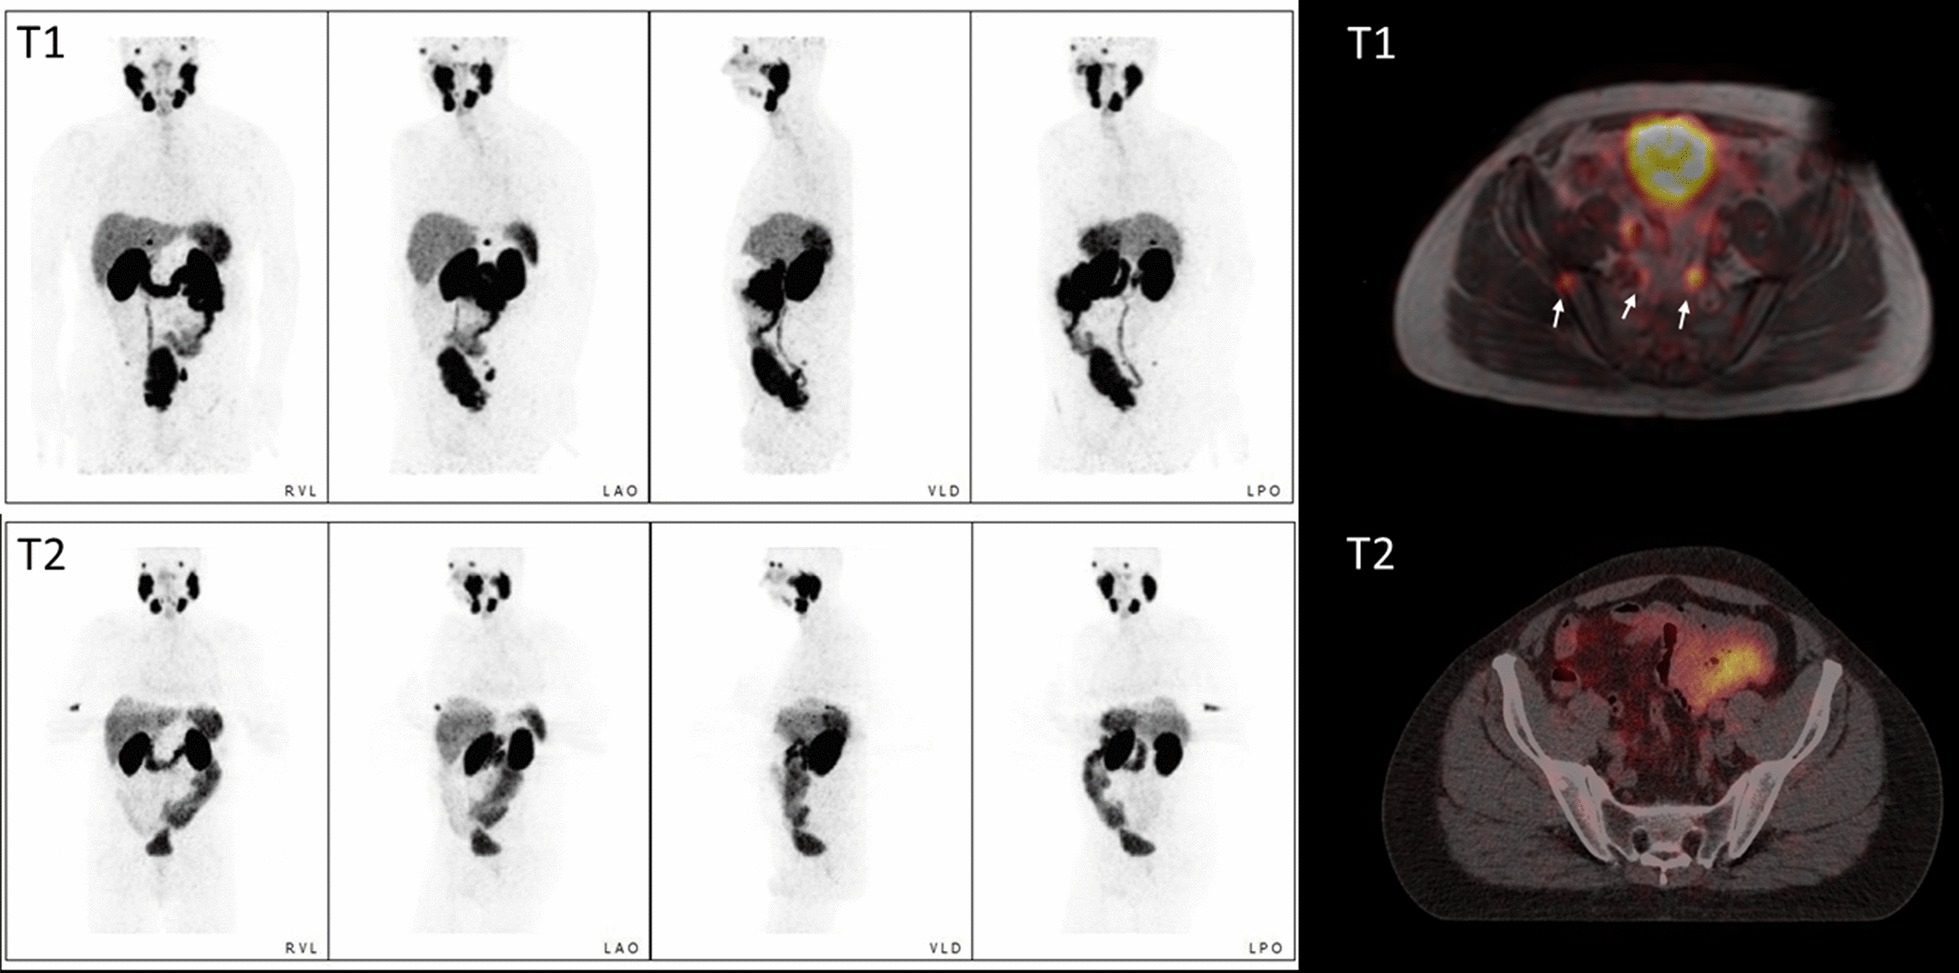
MIPs and fused PSMA PET/CT of Patient #3. The MIPs show a clearer demarcation of the prostatic tumor as well as the complete regression of lymphonodal metastases, whereas two new osseous metastases occurred (the lower one is indicated by the blue arrow)
